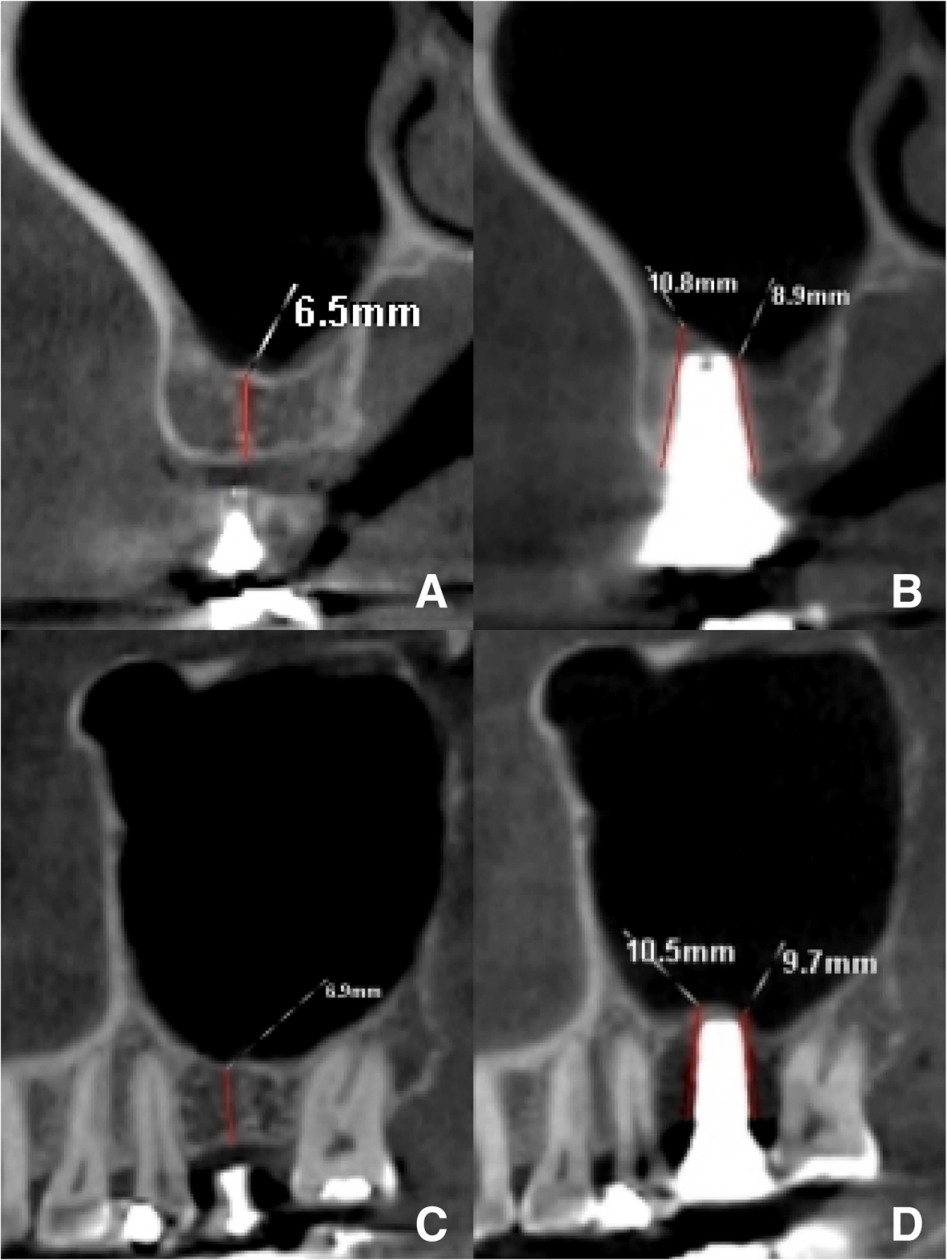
Case 1: The CBCT images of implant site 16 before (left column) and 6 months after surgery (right column). The residual bone height (RBH) was measured from the cortex bone to the alveolar bone crest under the sinus floor in a coronal and c sagittal views. The new bone level was measured from the most coronal implant thread to the most apical visible implant at b buccal and palatal sites in coronal view and d mesial and distal sites in sagittal view
